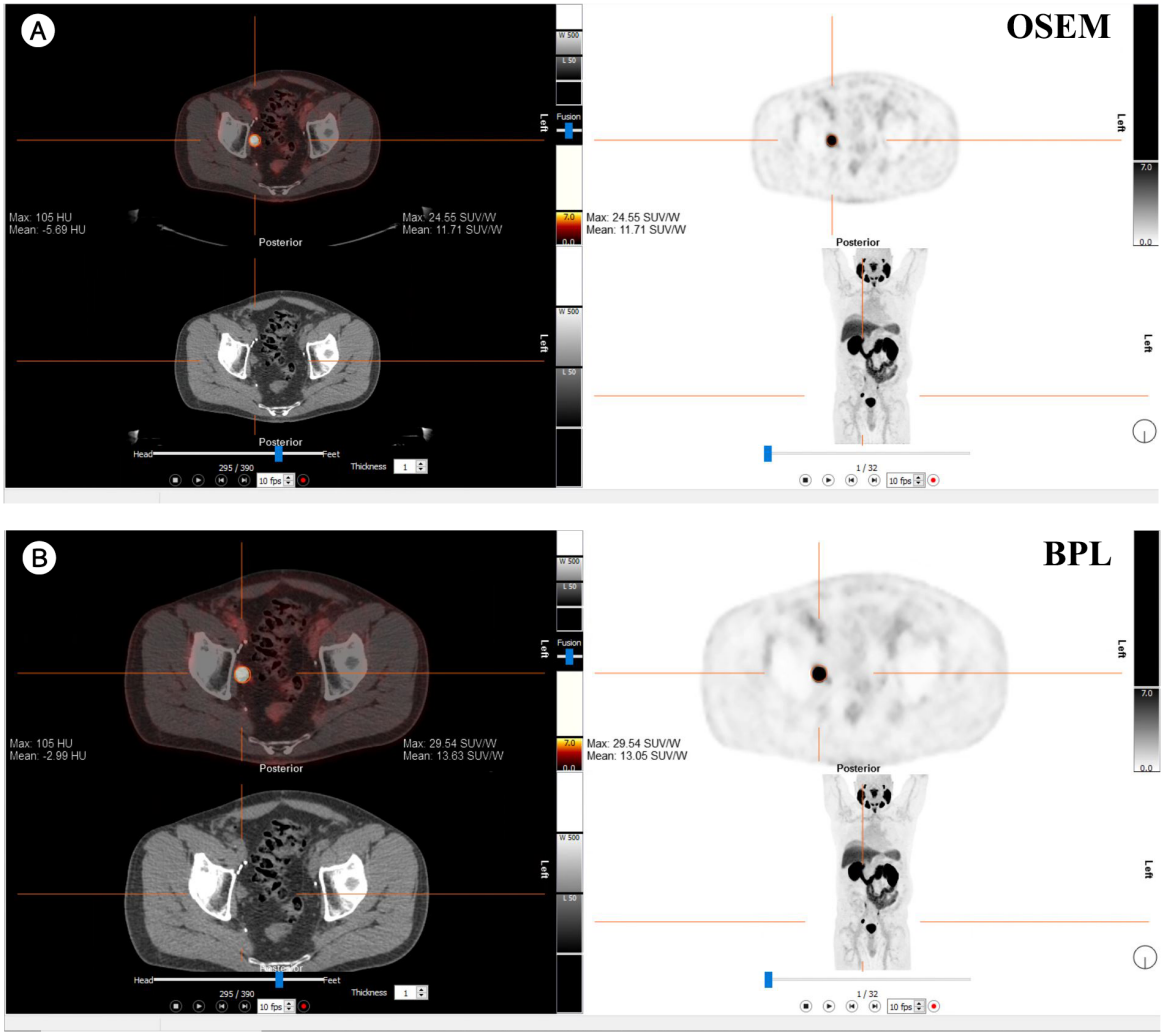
Comparison of PET/CT reconstruction results using OSEM and BPL algorithms with ^68^Ga-PSMA-11 in a patient with a right external iliac mass. (A) OSEM reconstruction; (B) BPL reconstruction. FDG, Fluorodeoxyglucose; OSEM, Ordered Subsets Expectation Maximization; BPL, Bayesian Penalized Likelihood; SUV, Standardized Uptake Value; BPL corresponds to Q.Clear (GE Healthcare).
